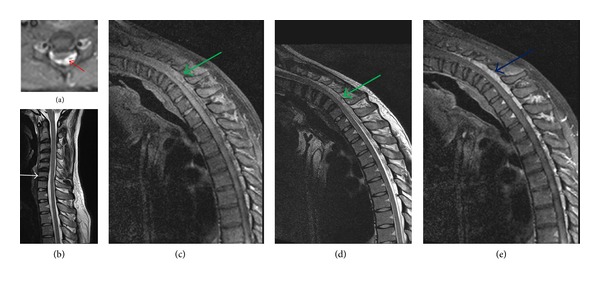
A 23-year-male with weakness and wasting of left hand and forearm with anteroposterior pear-shaped cord flattening with loss of attachment of dura from subjacent lamina (red arrow) on T2WI axial MRI (a), midsagittal image showing loss of cervical lordosis (white arrow) but not showing cervical cord atrophy on neutral position sagittal T2WI MRI (b), dorsal epidural voids (green arrows) on flexion sagittal T1WI and T2WI MRI ((c), (d)), and posterior epidural crescentic enhancing mass (blue arrow) on flexion contrast sagittal T1WI MRI (e).
